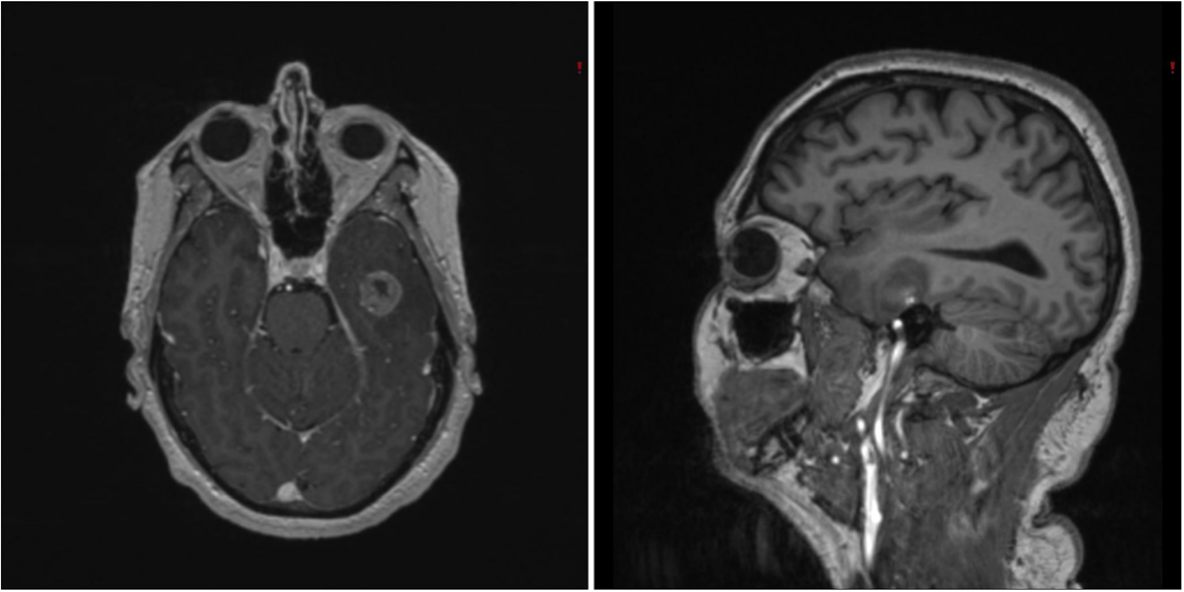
Magnetic resonance (MR)-imaging at initial evaluation at outside institution. T1-weighted post-contrast MR-imaging in the axial (*Left*) and sagittal (*Right*) planes demonstrating a contrast-enhancing lesion (maximum diameter 2.1 cm) that stereotactic needle biopsy confirmed to be glioblastoma multiforme. After biopsy, the patient underwent laser interstitial thermal therapy of the enhancing tumor
